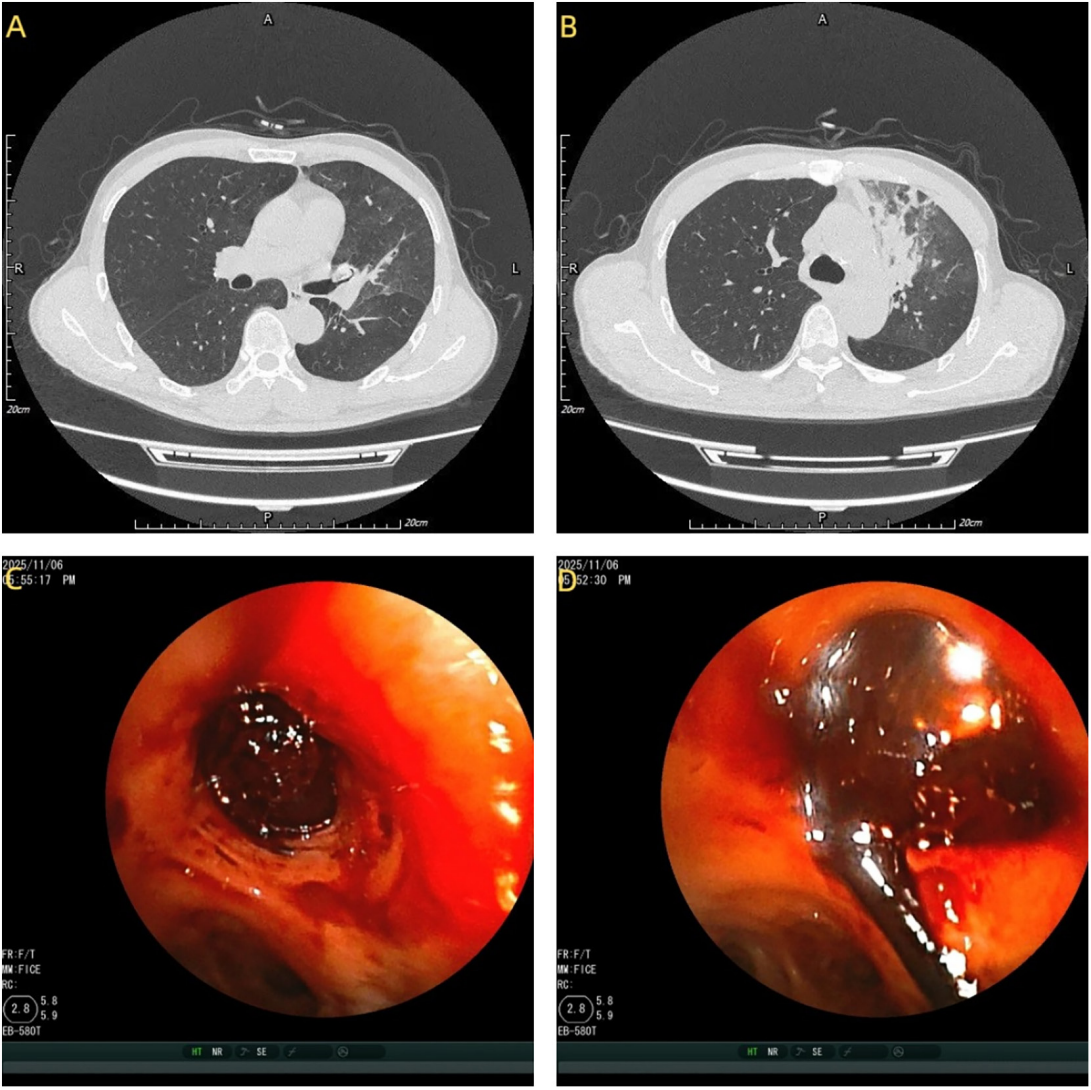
(A, B) CT of the thorax showed the consolidation of the left upper lobe with obstruction of the segmental and subsegmental bronchi in both the left upper and lower lobes; (C, D) fibreoptic bronchoscopy showed the left upper lobe was completely obstructed by jelly‐like thrombi.
